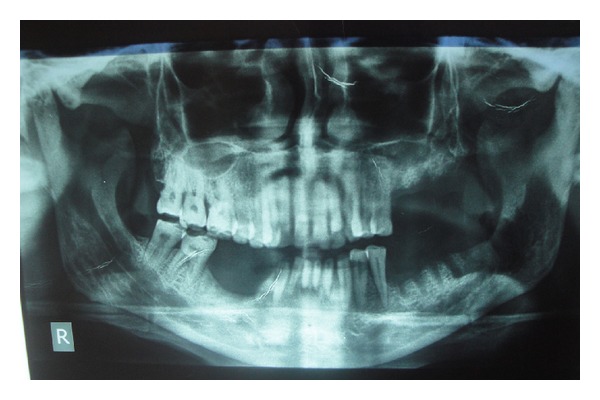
Orthopantomogram (OPG) revealed ill-defined osteolytic region involving body and ascending ramus of mandible.
